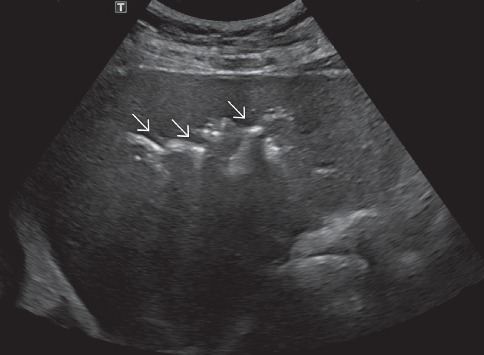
Abdominal ultrasound demonstrates the appearance of pneumobilia: bright echogenic foci in linear configuration (arrows) with posterior acoustic shadowing and reverberation artifacts.
